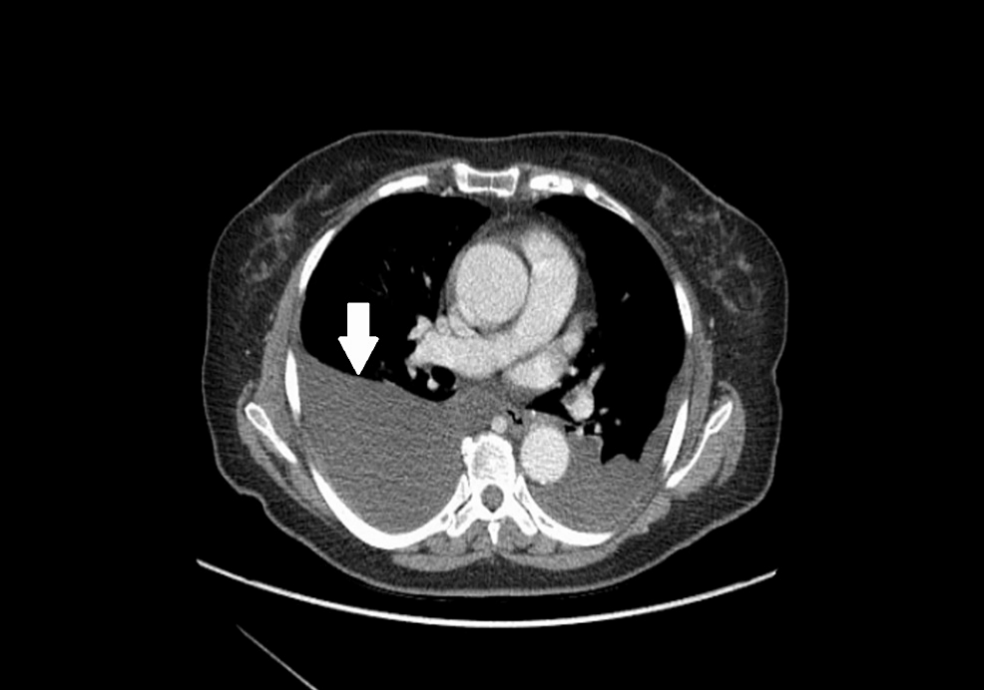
CT scan of the chest: axial view showing right pleural effusion greater (white arrow) than left.
